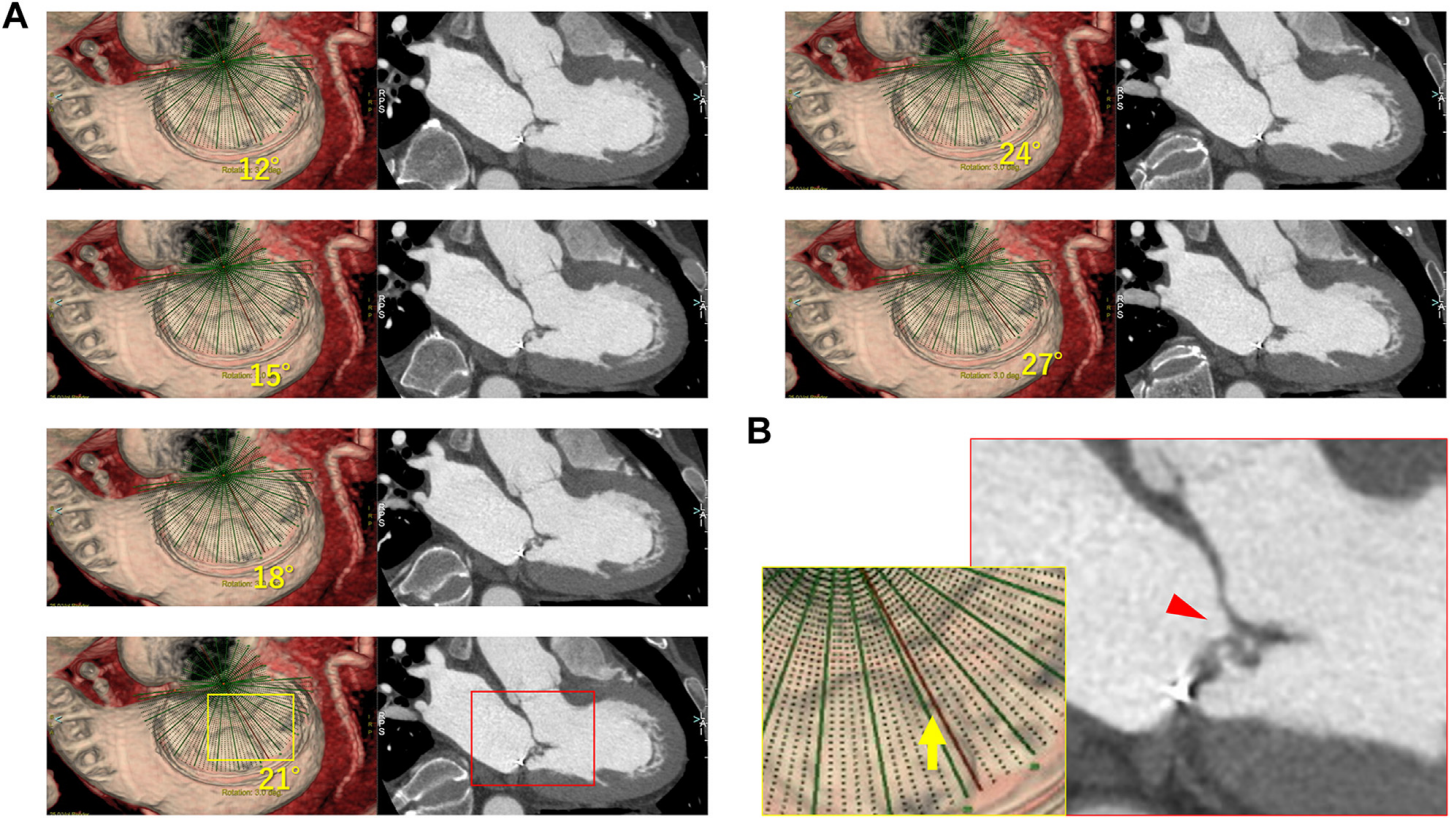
Postoperative computed tomography findings in mitral valve–radial sectional view. (A) Continuous images at 12° to 27° every 3°. (B) Enlarged views of the areas marked with yellow and red squares in the image at 21° in (A). The yellow arrow indicates the indentation between P2 and P3, and the red arrowhead clarifies a gap between the anterior posterior leaflets.
